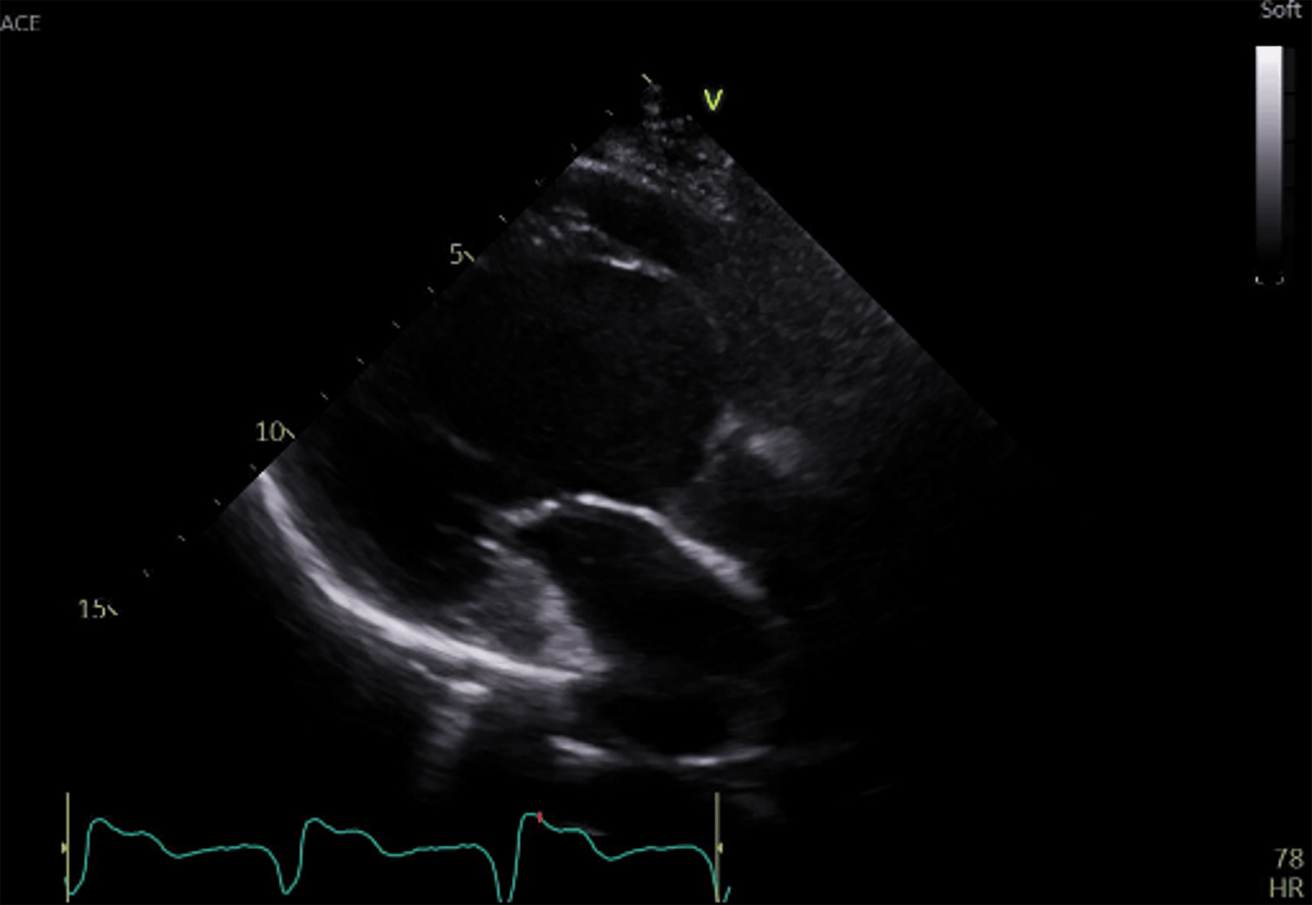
Preoperative echocardiographic findings. Left ventricular systolic dysfunction was observed, with a left ventricular ejection fraction of 14% (Teichholz method).
